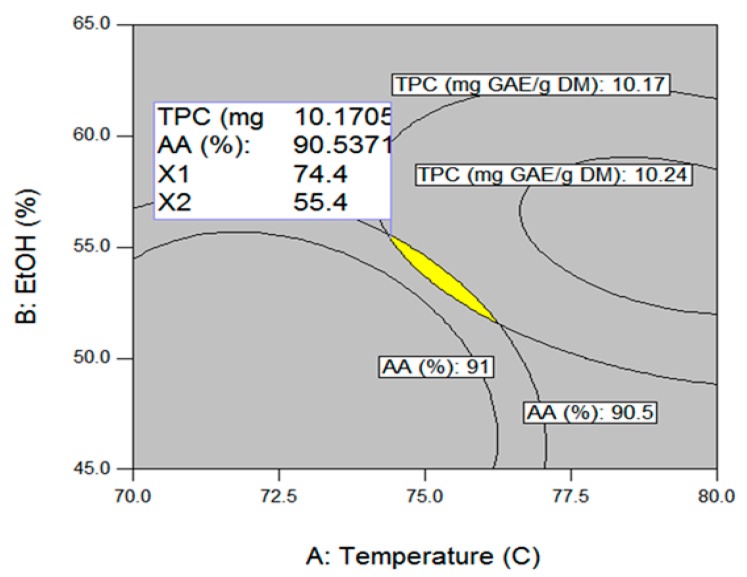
Optimization of extraction of total phenolic compounds and antioxidant activity based on the superimposing method. The interactive effect of extraction time and ultrasonic power on total phenolic compounds and antioxidant activity at constant levels of extraction time of 59.6 min, and ultrasonic power of 700 W.
